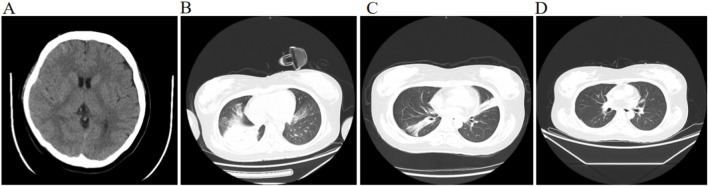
Head and Chest CT scan during hospitalization (A–D). Head CT upon admission (A). Chest CT upon admission (B). Chest CT 2 weeks post-admission (C). Chest CT 2 weeks post-discharge (D).
